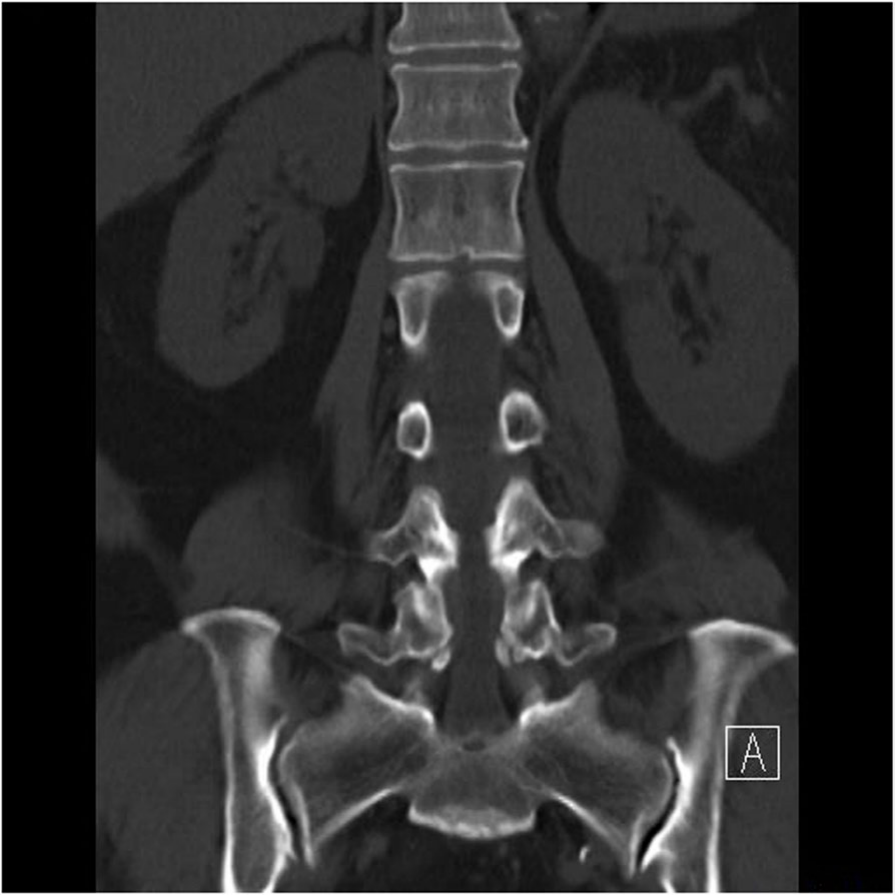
The coronal view of CT effectively reveals the cranial lateral recess spur configuration
